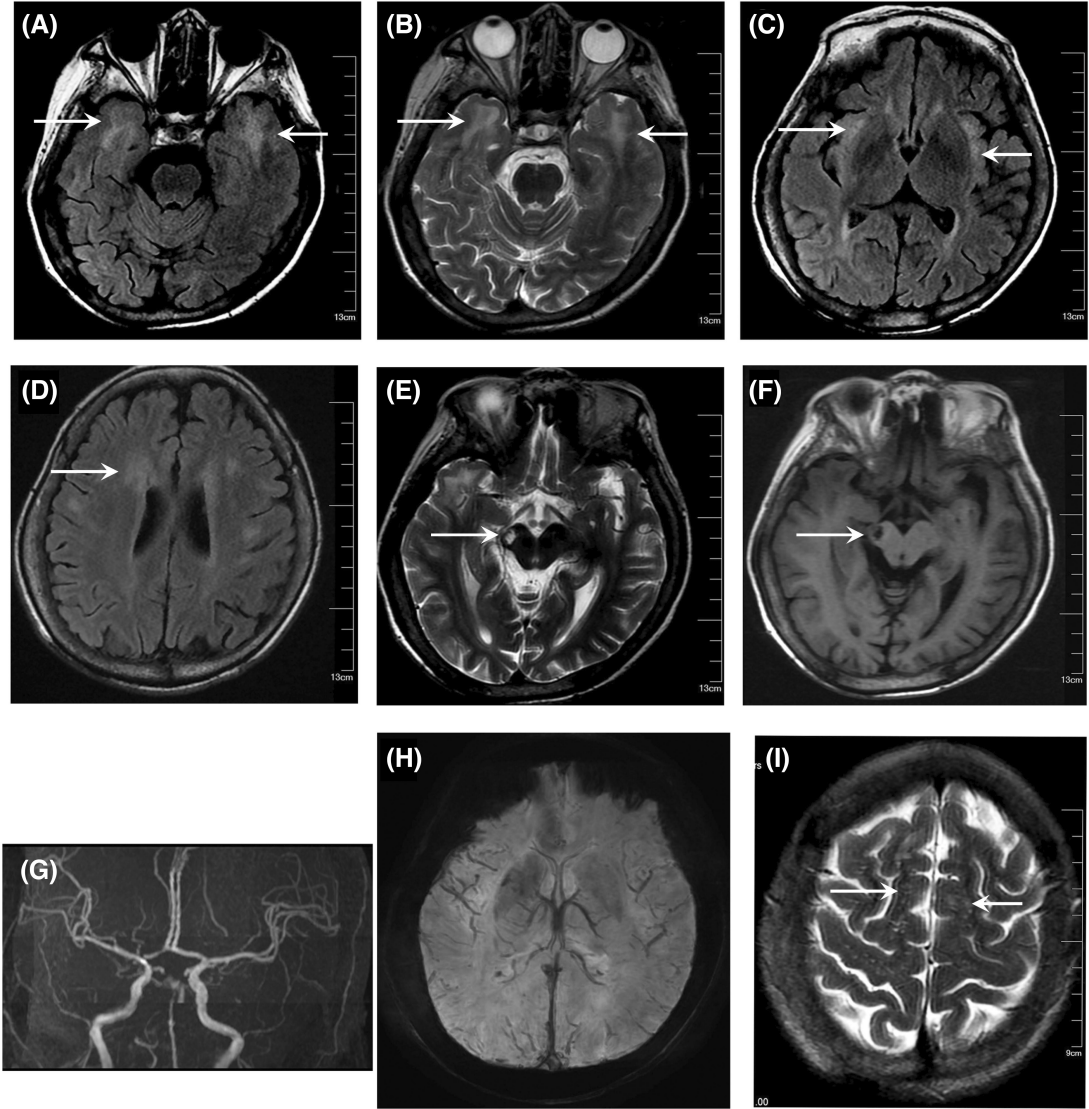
MRI images of this patient. Axial FLAIR (A) and T2‐weighted (B) images at the same level show white matter lesions in the temporal lobes. Axial FLAIR‐weighted images (C and D) show confluent white matter lesions in all cerebral lobes and signs of brain atrophy. Cerebral infarction in right cerebral peduncle 3 months ago (E and F). MRA show basilar artery arteriosclerosis (G). Axial susceptibility weight‐imaging (SWI) images showing the absence of microbleeds (H). Axial T2‐weighted image shows prominent Virchow‐Robin spaces in the centrum semiovale (I)
